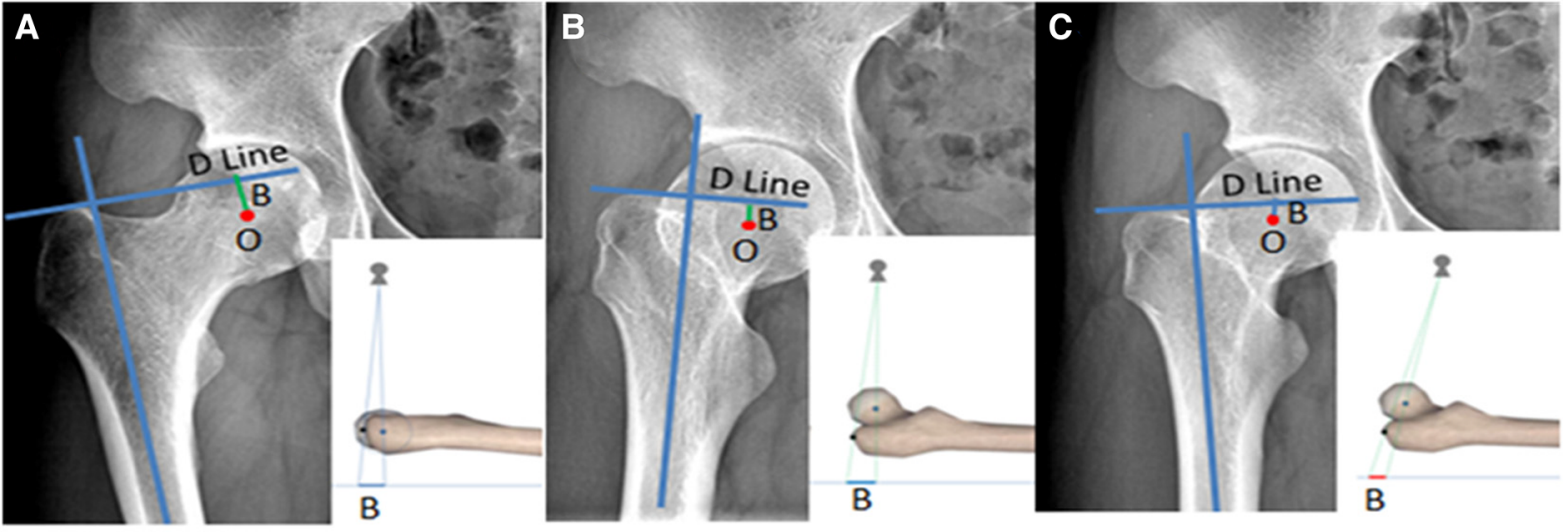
Bilateral hip x-ray of the same volunteer: (A) is the standard photographic method as previously mentioned, (B) is the externally rotated hip and the projection point is standard, and (C) is the externally rotated hip and the projection point is 10 cm below the midpoint of the bilateral hips; the femoral head center is significantly higher than the actual value shown in (A).
